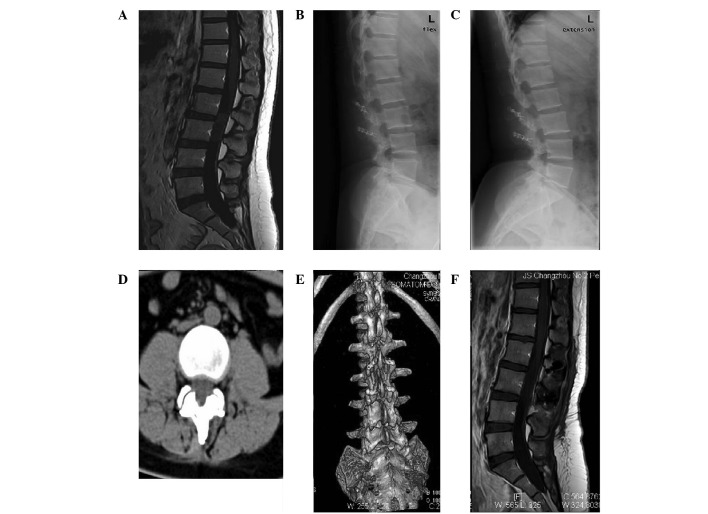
Case 2. Ependymoma at the L2–3 level. (A) Prior to surgery, magnetic resonance imaging (MRI) identified that the L2–3 spinal space was occupied by the tumor. (B and C) One year following the surgery, X-ray examination indicated no fixation transposition or fracture, lumbar instability or kyphosis. Three months following the surgery, favorable lamina bone fusion was indicated by (D) a postoperative computed tomography (CT) scan and (E) 3-D reconstruction. (F) Six months following the surgery, no intraspinal scar adhesions or restenosis were identified by MRI.
